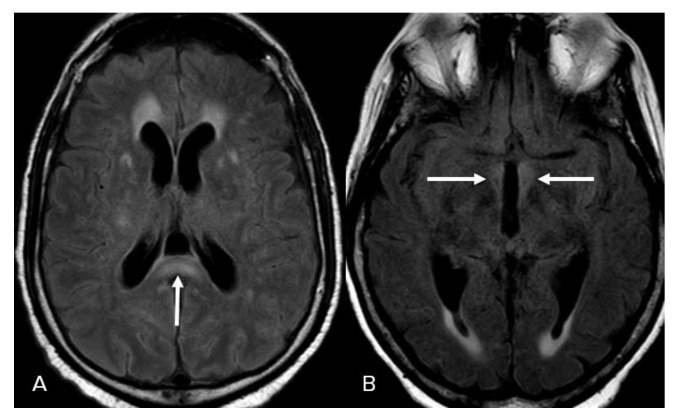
Less common patterns. (A) Axial FLAIR images show FLAIR hyperintensity of the splenium of the corpus callosum. (B) FLAIR hyperintensity around the third ventricle. Image has been windowed manually to improve the visibility of signal abnormality.
